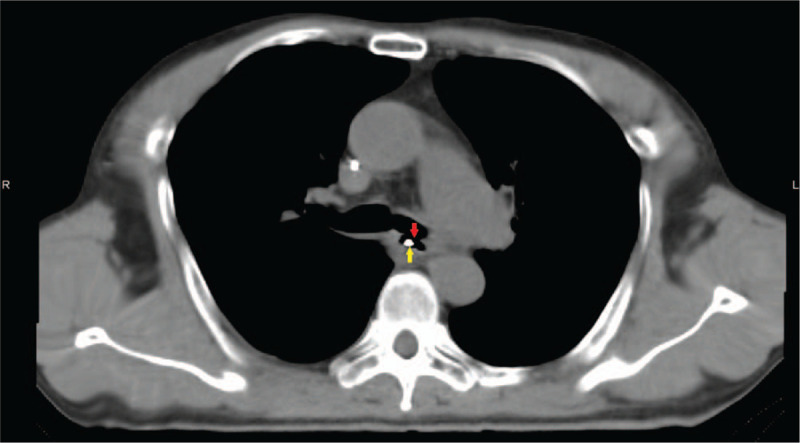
A chest computed tomography scan showed a tracheoesophageal fistula (red arrow) and jejunal feeding tube (yellow arrow).
